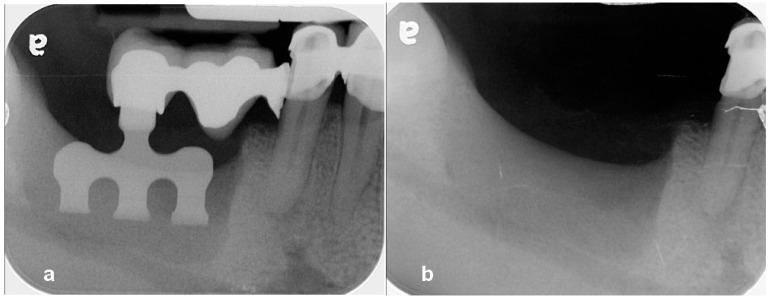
(a) A periapical radiograph revealed fracture restoration and severe bone resorptionaround the blade implant. (b) Note the severe three-dimensional bone defect after removal of the implant.
